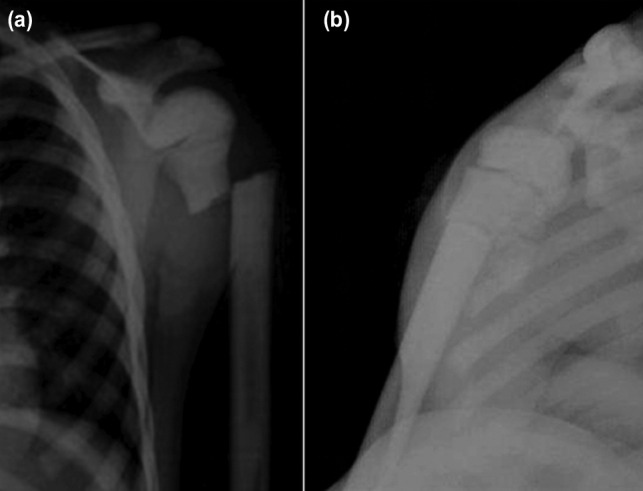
(a) Anteroposterior and (b) transthoracic lateral shoulder radiographs of the patient at initial admission. Note the pattern of fracture (transverse) and the diffuse sclerosis of humeral bone without an intramedullary canal.
